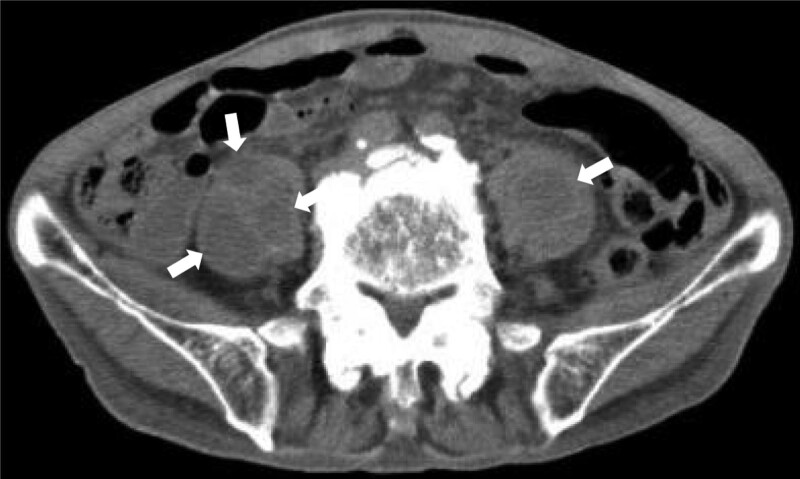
The abdominal computed tomography (CT) at lower level of 5th lumbar in case 1 of series 1. The arrows show multiple abscesses in bilateral psoas muscles.
